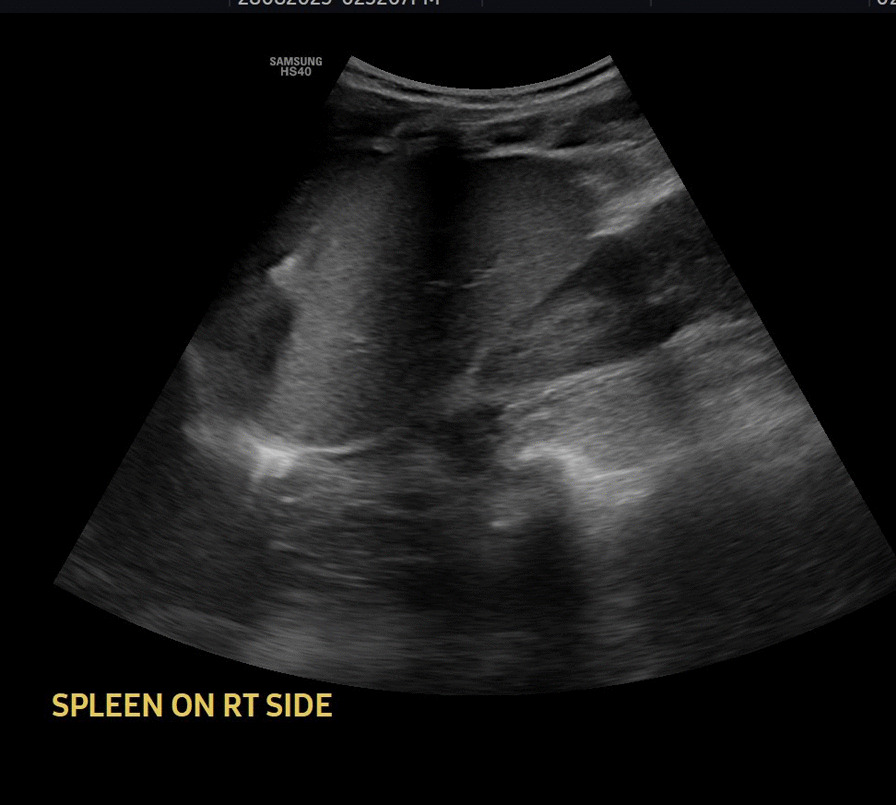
Sonographic picture of normal spleen situated on the right side
